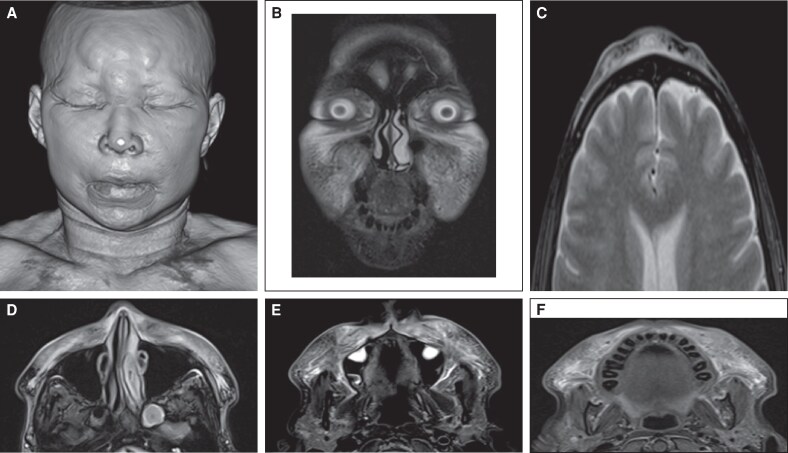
MRI of the paranasal sinuses and facial region using a T_2_-weighted fat-suppressed sequence in coronal and axial sections. In a 58-year-old woman with a history of nonabsorbable exogenous substance injections, diffuse edema of the skin and subcutaneous tissue is observed without fluid collections in the following areas: (A) volumetric facial reconstruction; (B) facial region; (C) frontal fat; (D) nasal and zygomatic regions, with mucosal opacification containing high-protein material in the left sphenoid sinus; (E) nasolabial region; and (F) perioral space, maxillary fat, and retromaxillary fat.
